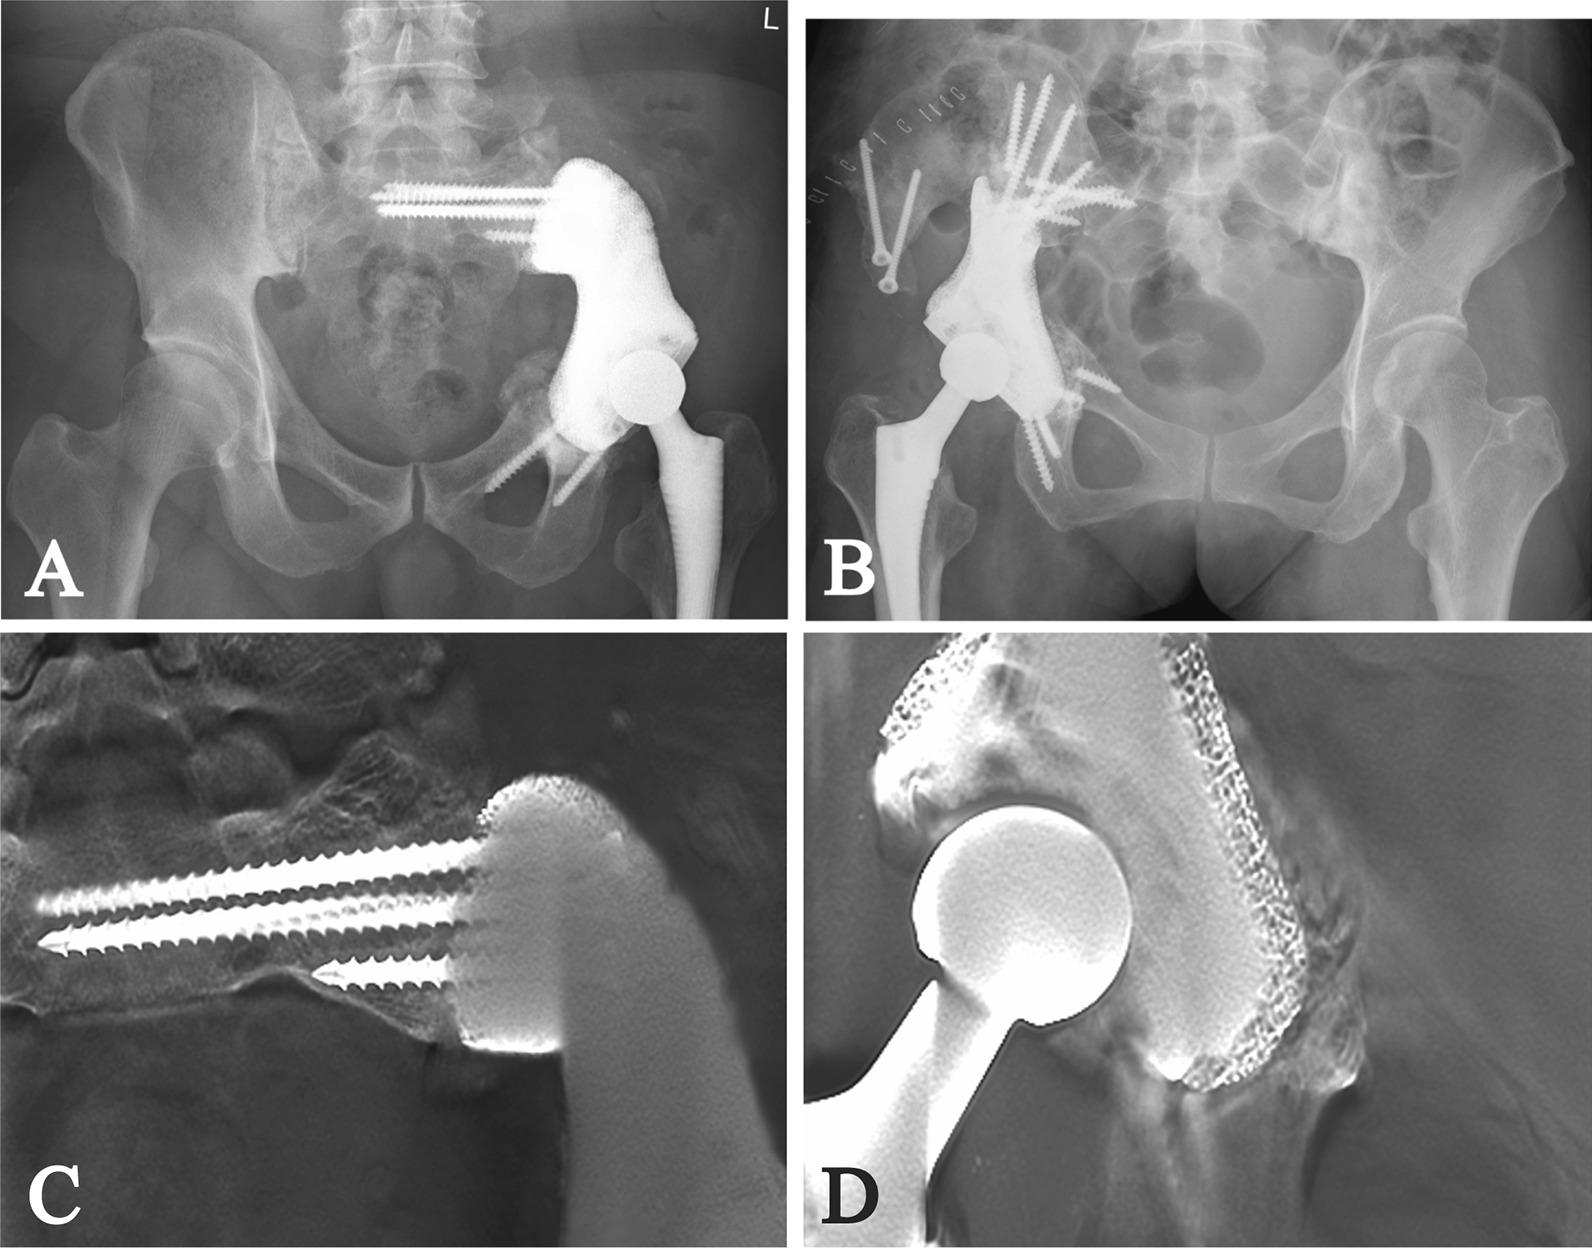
A, B Two AP plain radiographs of (A) patient 1 and (B) patient 2 after implantation of custom-made hemipelvic endoprosthesis show planned reconstruction is obtained. C, D The digital tomosynthesis images show ideal osseointegration in (C) patient 1 and (D) patient 2
